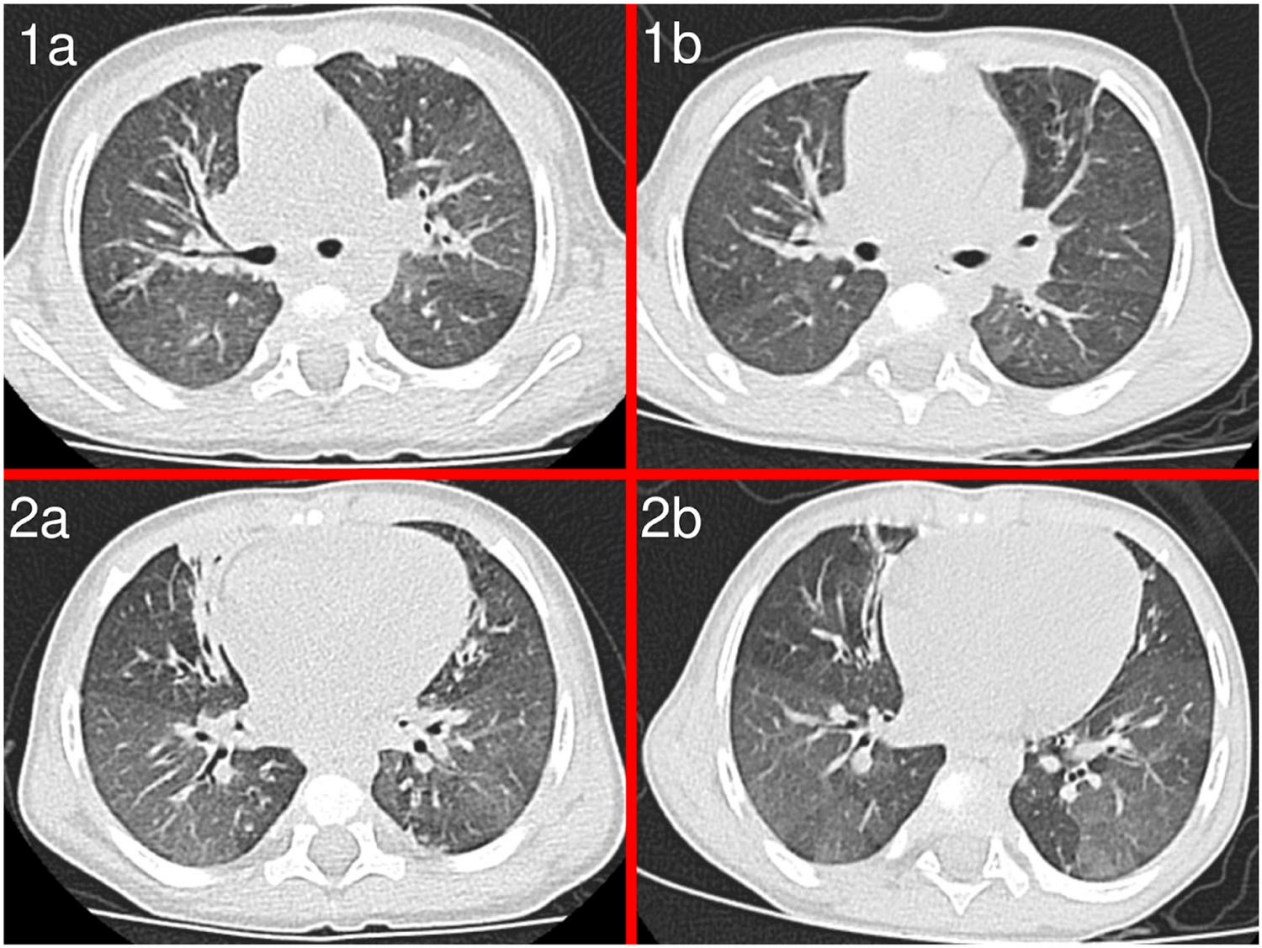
CT findings (patient 2). 1‐2a: CT scans before steroid treatment, showing peri‐bronchial inflammation, mosaic perfusion features and air trapping areas. 1‐2b: CT scans after steroid cycles, documenting a reduction of peribronchitis with unchanged mosaic perfusion pattern. [Color figure can be viewed at wileyonlinelibrary.com]
